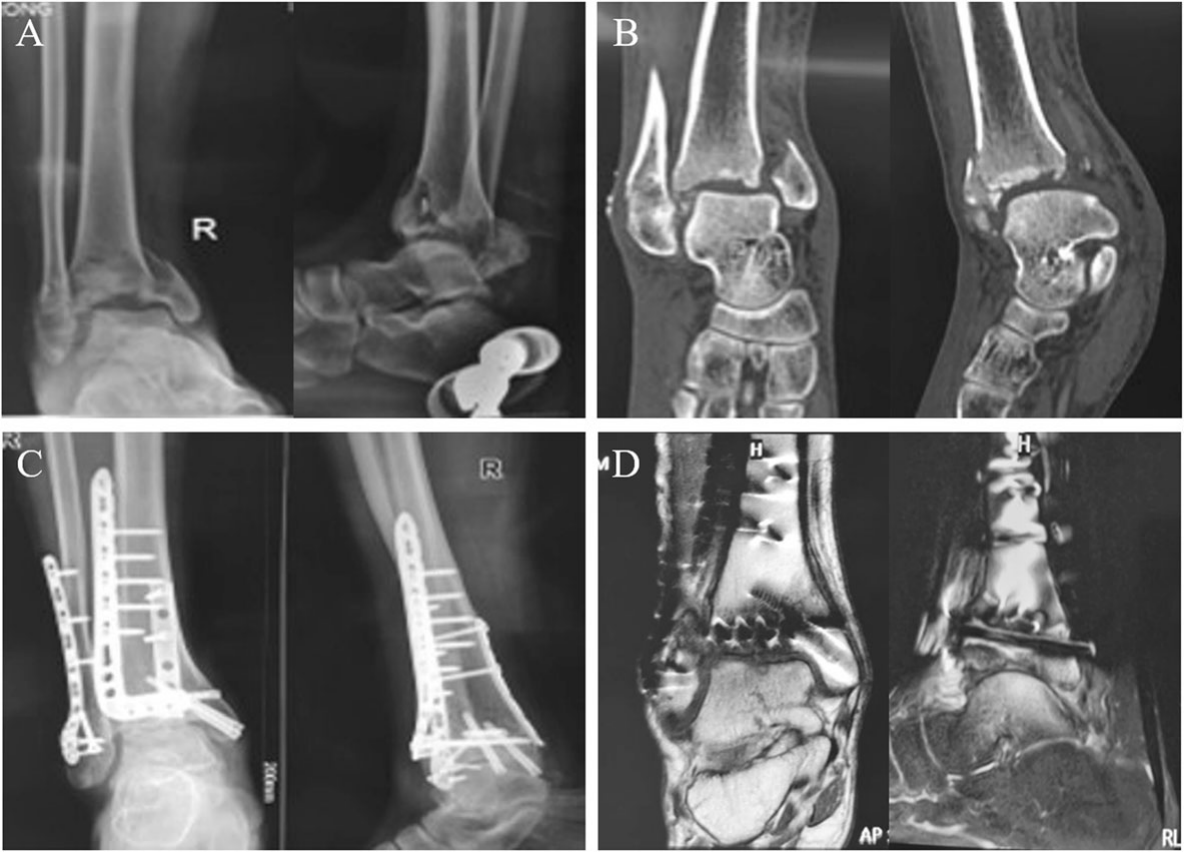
Healing progress of a typical case following reconstruction surgery. a, b Pre-operative radiograph and CT images of a 19 year old male patient. c Post-operative radiograph of the patient at 18 months follow-up. ds MRI images showing the integrity and surface of the reconstructed tibiotalar joint
